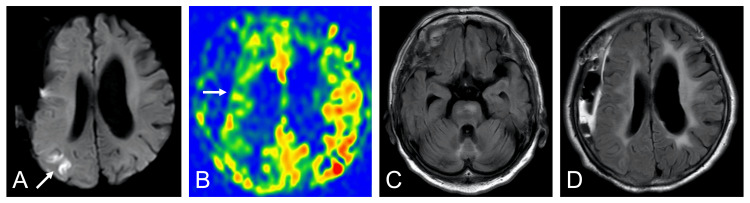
Magnetic resonance imaging Magnetic resonance imaging on postoperative day 4 showing a right parietal infarct on diffusion-weighted imaging (A) (arrow), decreased cerebral blood flow signal in the right middle cerebral artery territory on arterial spin labeling imaging (B) (arrow), and no subarachnoid hemorrhage on fluid-attenuated inversion recovery imaging (C, D)
